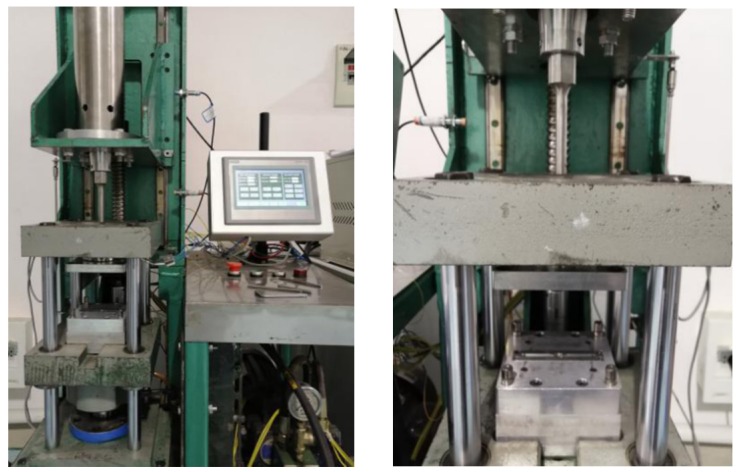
Prototype machine for ultrasonic plasticization.
